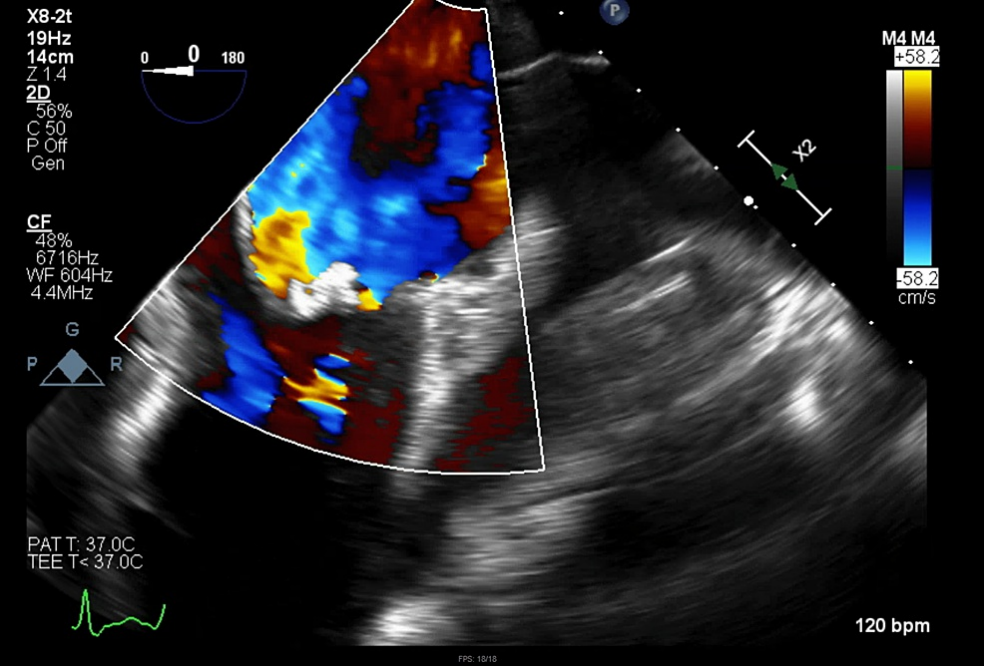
Transesophageal echocardiogram (TEE) showing mitral regurgitation.
